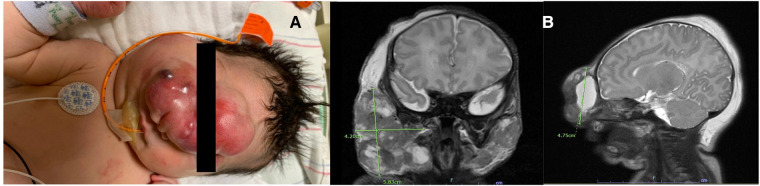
(A) Image of the infant's face taken on presentation demonstrating numerous facial lesions, particularly large midline face mass. The mass extended centrally from her forehead, down the length of her nose, to the midway on her right cheek, obscuring the right eye completely and the left eye partially. An additional large round mass was located above her right parotid gland with no extension noticed in her mouth, including the hard palate. (B) Initial MRI of the brain/face showing lesions predominately in the midline of the infant's face as well as the right parotid gland.
